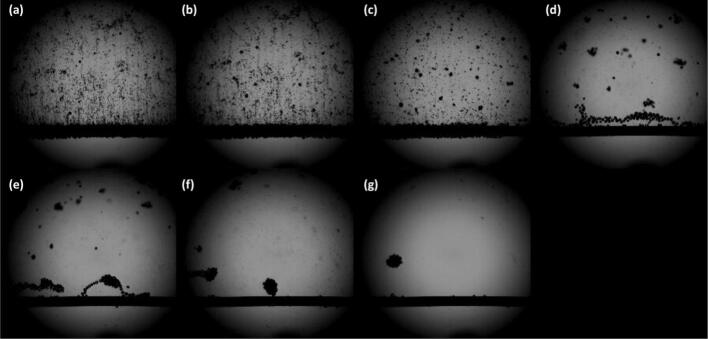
Hydrogen generation on a Pt wire in the absence (a) and presence of ultrasonication (26 kHz, 75 W/cm^2^, (b)-(g)). The applied potential was set at − 1.30 V/RHE. (a) 0 μs, (b) 100 μs, (c) 200 μs, (d) 300 μs, (e) 400 μs, (f) 500 μs, (g) 600 μs. The time between each image is 10^-4^ s (100 μs) filmed at 10,000 frames per second [44].
